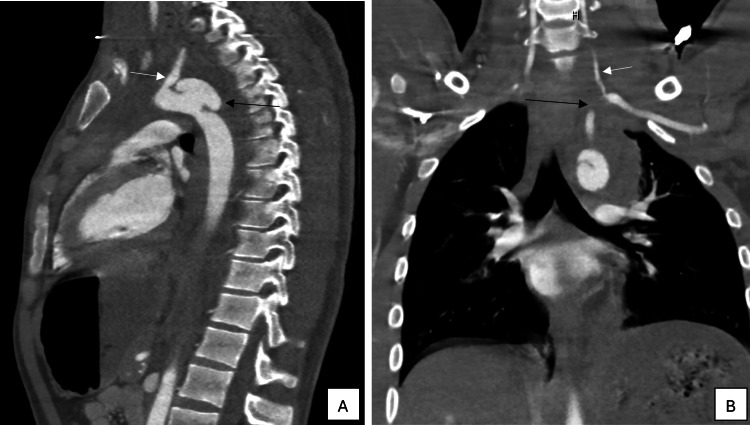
A: Saggital view CT angiogram of the thoracic aorta. B: Coronal view CT angiogram of the thorax. *A:* Large pseudoaneurysm (long black arrow) arising from the aortic arch juxtaductal to the left subclavian artery (short white arrow).* B:* Left proximal subclavian artery occlusion (long black arrow) with distal opacification by the left vertebral artery (short white arrow).
